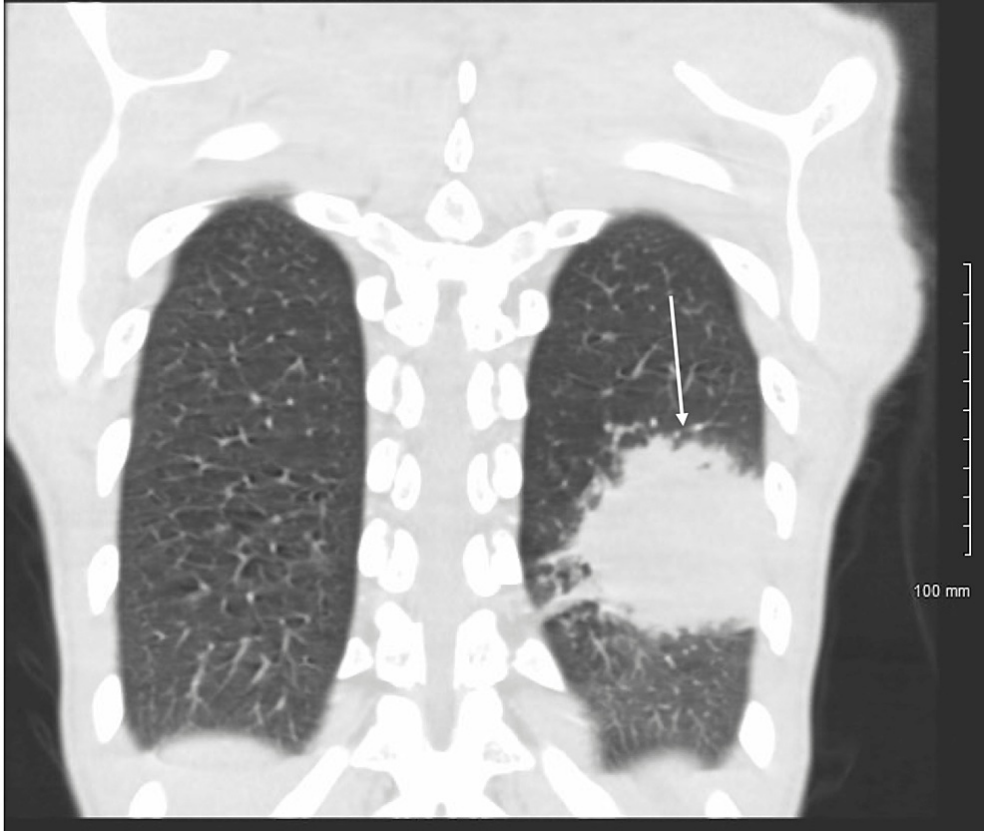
Coronal section of a computed tomography slice showing the lung mass in the left lobe
